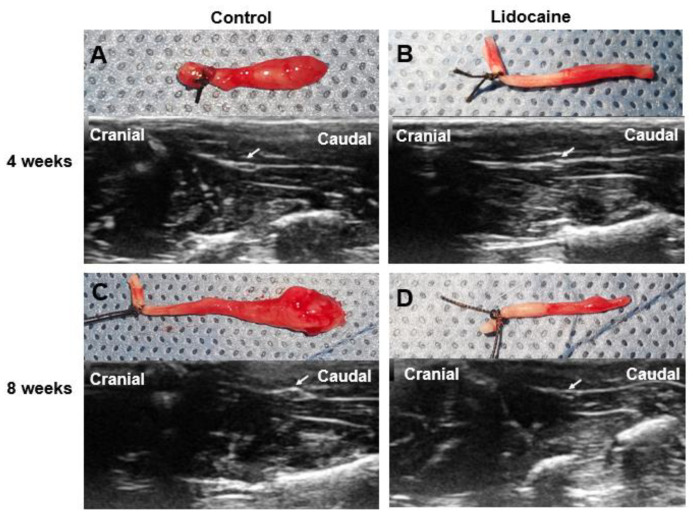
Images of the typical proximal segment of a severed sciatic nerve and its ultrasound images in two groups at 4 weeks and 8 weeks after neurectomy. A bulbous neuroma in the proximal stump was observed in the saline control group (A,C), while no obvious neuroma was seen in the lidocaine control group (B,D). The white arrow indicated the proximal end of the nerve.
